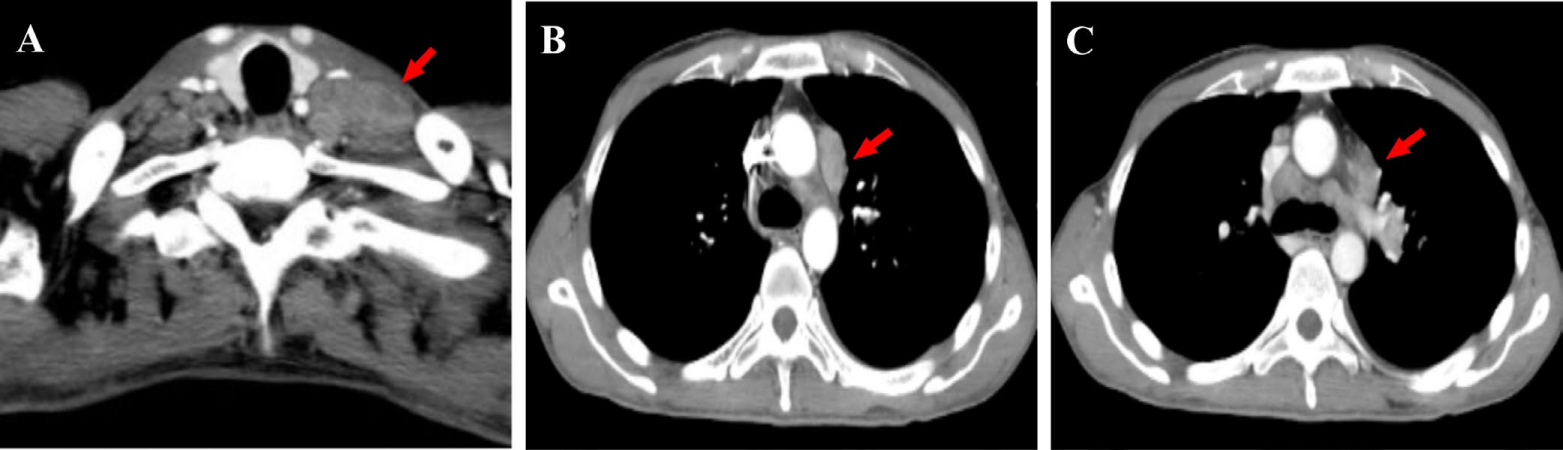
CT examination results. (A) The CT scan revealed the largest lymph node mass measuring approximately 30 × 15 mm located above the left clavicle (indicated by the red arrow); (B) A mass was observed adjacent to the aortic arch (indicated by the red arrow); (C) A mass was identified near the left hilar region of the lung (indicated by the red arrow).
